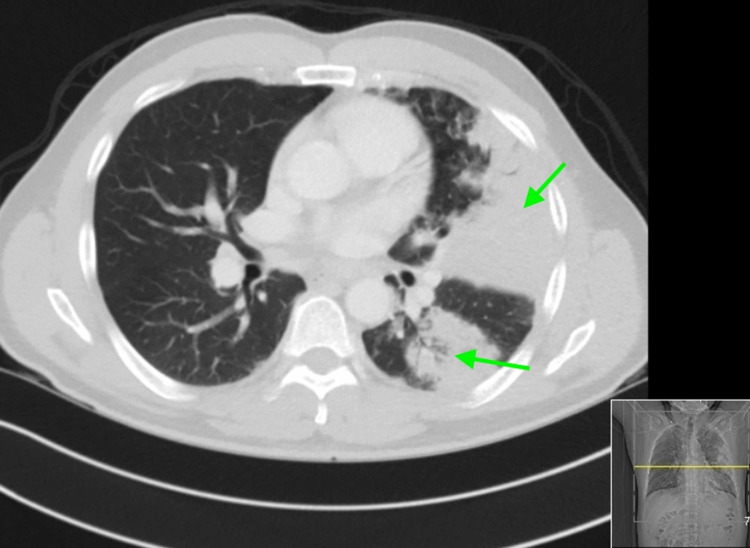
CT of the chest - image 3 The image shows dense areas of pulmonary consolidation particularly involving the left upper lobe with the consolidation of at least half of the left upper lobe (arrows) CT: computed tomography
